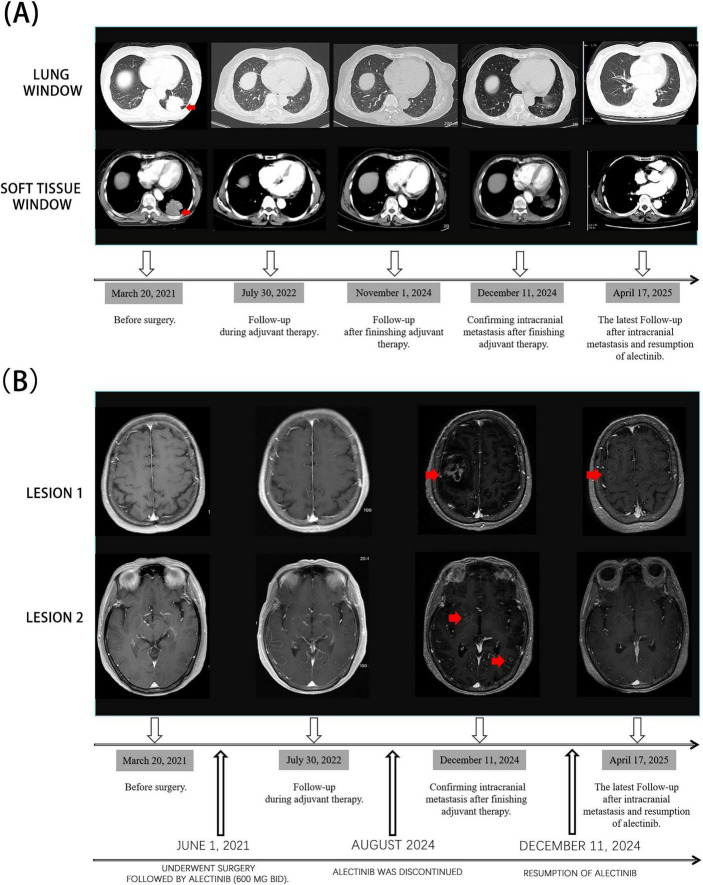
Changes in tumor burden during the patient’s treatment. (A) Lung and soft-tissue windows on contrast-enhanced chest CT during treatment. (B) Brain contrast-enhanced T1-weighted MRI during treatment.
